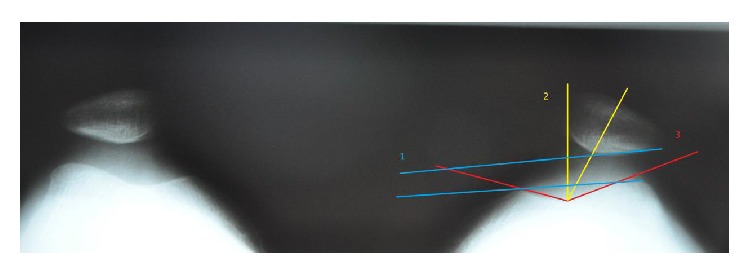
Preoperative X-rays according to Merchant view in 45° of flexion with quadriceps tension. (1) Patellofemoral angle. (2) Congruence angle. (3) Sulcus angle.
